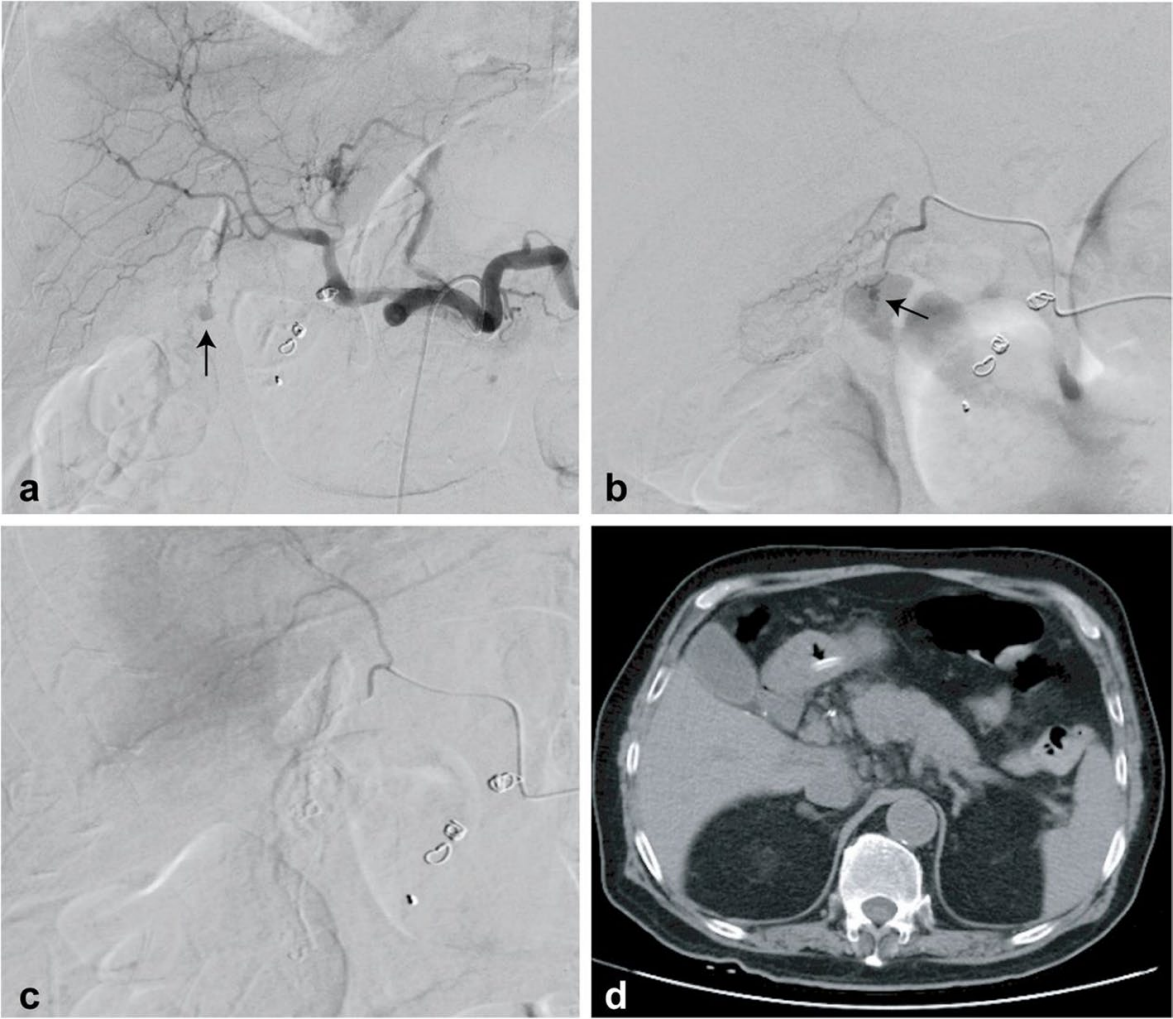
Case 2. a Angiography after empirical gastroduodenal artery embolization shows contrast extravasation (arrow). b Super-selective angiography confirms the source of bleeding is the cystic artery (arrow). c The cystic artery is embolized with a mixture of NBCA and iodized oil in a 1:4 ratio. d One month post-procedural CT scan shows no gallbladder infraction
